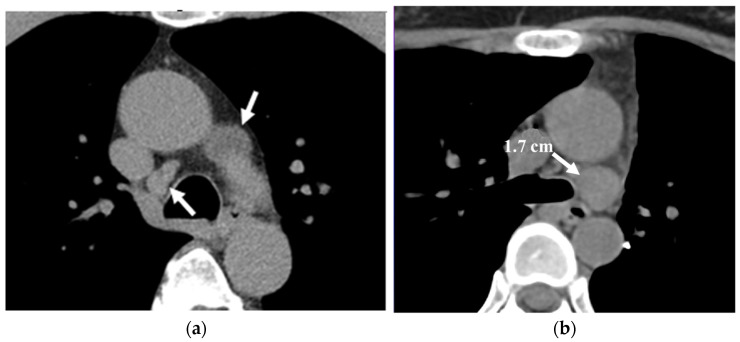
Mediastinal lymph nodes (LNs) in a low-dose computed tomography (LDCT) scan for lung cancer screening. (a) An axial LDCT image of a 61-year-old male shows nodes, with each measuring less than 15 mm in short-axis diameter (arrows), typically requiring no further workup. (b) An axial LDCT image of a 40-year-old female showing a node measuring 1.7 cm in short-axis diameter (arrow), warranting surveillance or a follow-up contrast-enhanced chest CT within 3–6 months in the absence of an identifiable cause.
